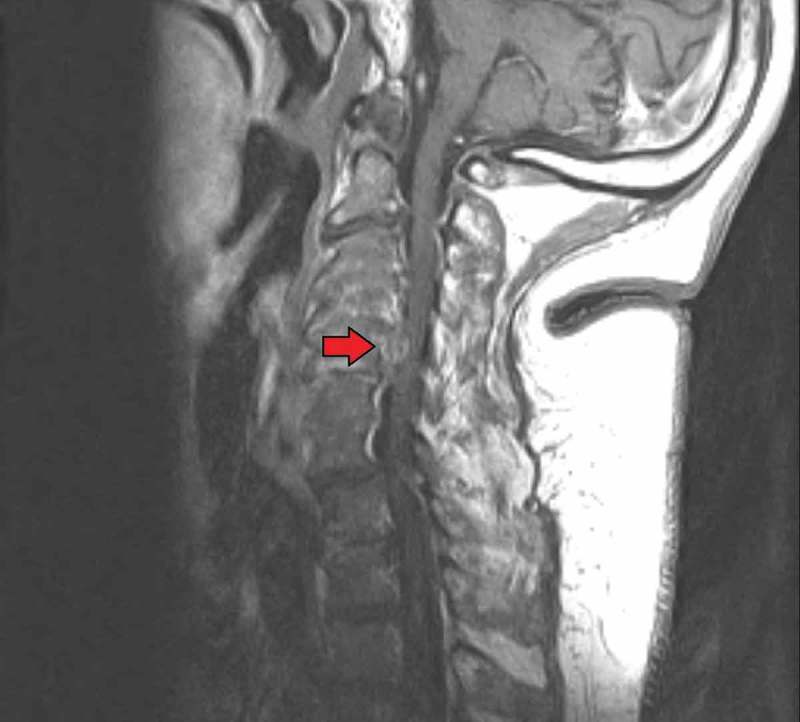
Sagittal section of cervical spine magnetic resonance image (MRI) showing mass on the inferior border of the C5 epidural space.
